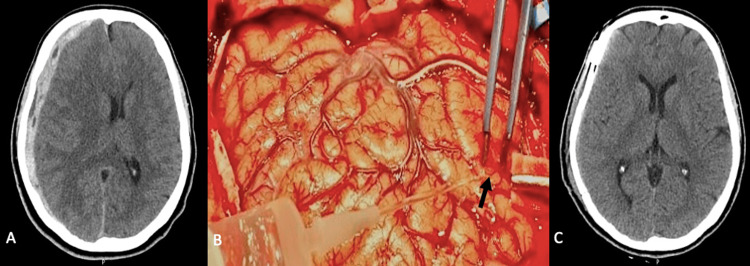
(A) Head CT scan with ASDH and midline shift. (B) Cortical microaneurysm (arrow). (C) Postoperative head CT scan. CT, computed tomography; ASDH, acute subdural hematoma
